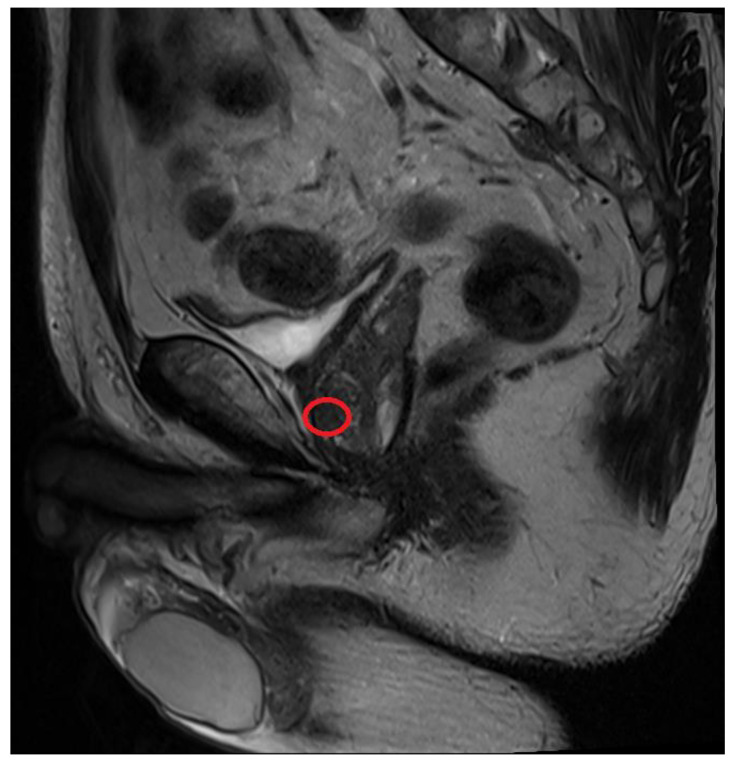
Suspicious lesion on the sagittal plane of T2-weighted image. The lesion was located at the apex of the prostate (red circle).
